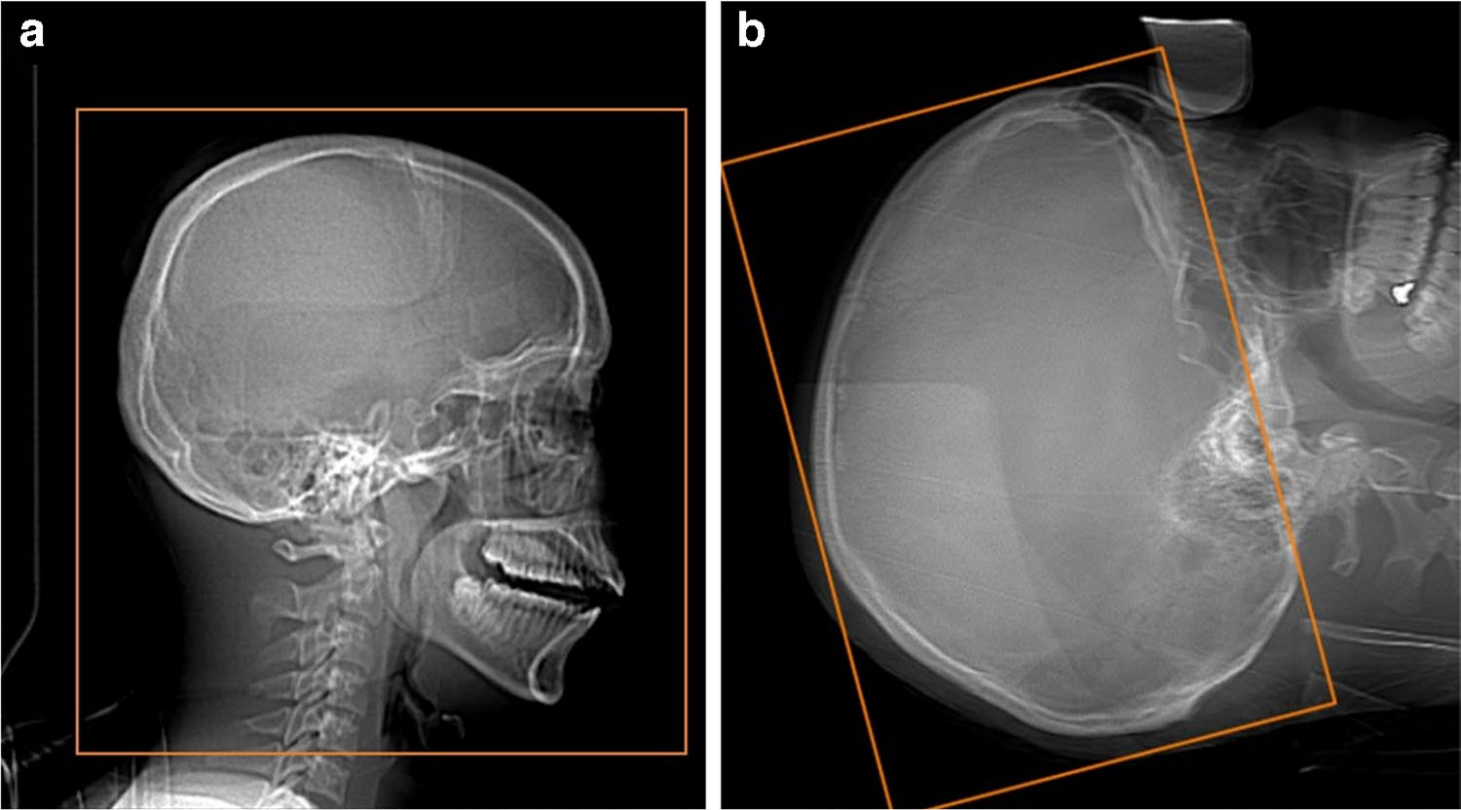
A computed tomography scan in the trauma setting without (a) and with (b) gantry tilting
